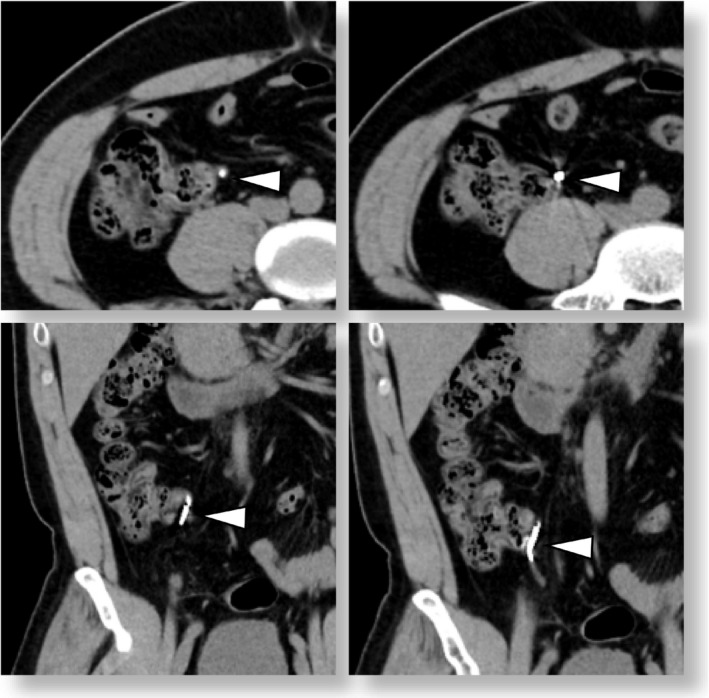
Abdominal computed tomography
